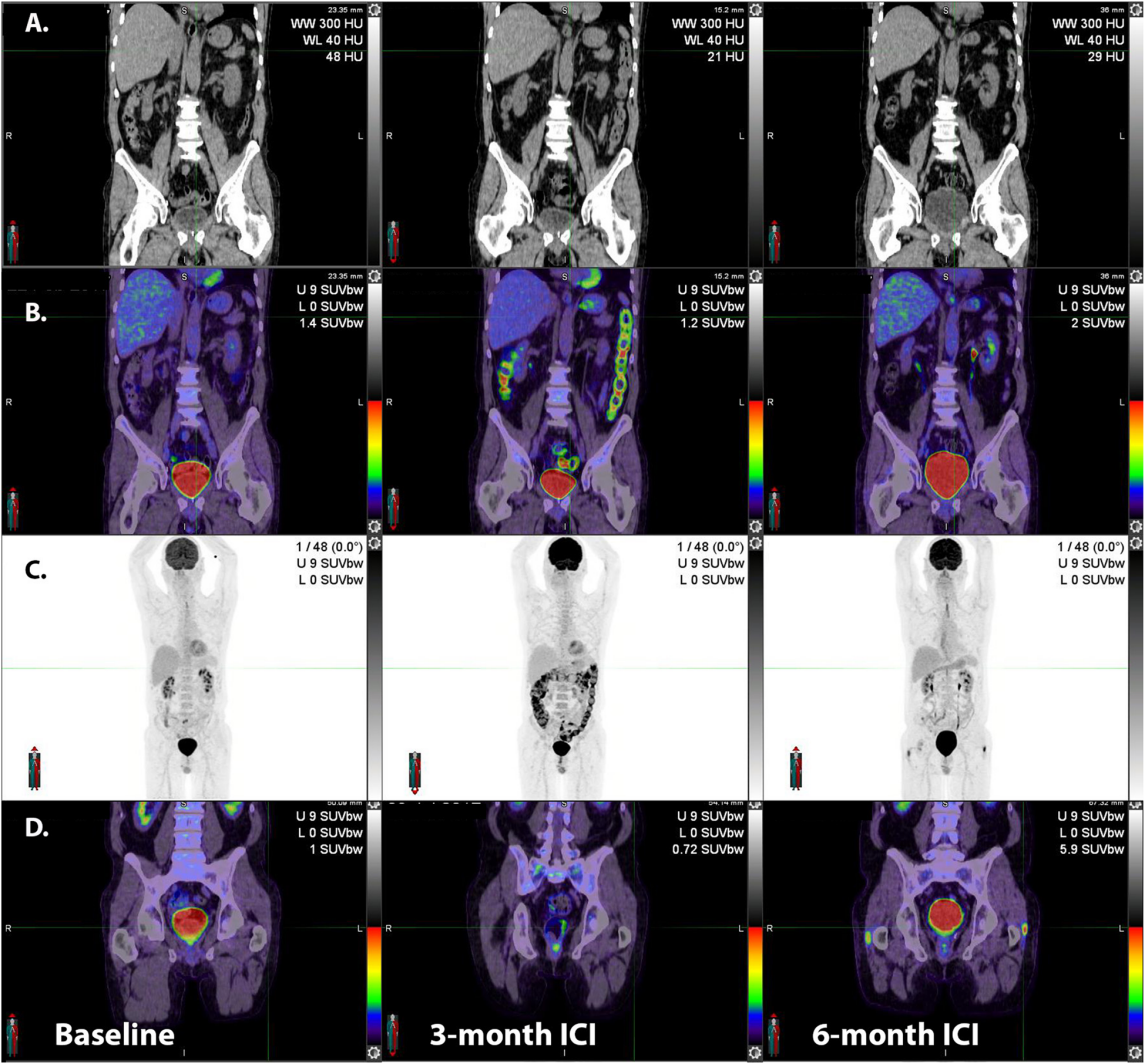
Following the introduction of adjuvant immunotherapy for resected stage IIIC disease, despite normal CT appearances (A, middle panel), fused ^18^F-FDG PET/CT (B, middle panel) and MIP (C, middle panel) images revealed intense uptake throughout the large bowel with a typical “string of pearls” distribution. The patient was asymptomatic at the time of the scan but within 24-h had developed severe diarrhea consistent with this diagnosis. Despite successful treatment of this complication the patient subsequently developed bilateral trochanteric bursitis as a second potential irAE
